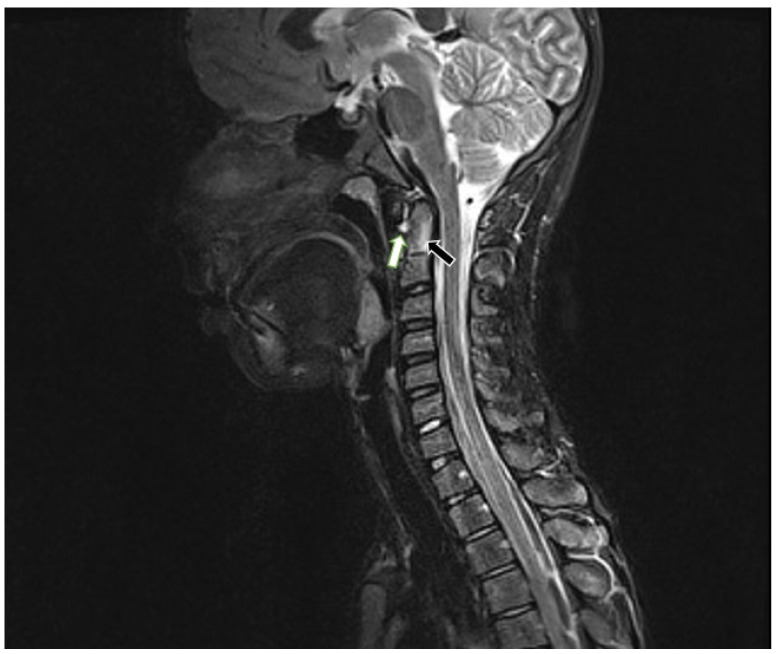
Sagittal MRI, TIRM T2w sequence in a 12-year-old boy diagnosed with enthesitis-related arthritis shows BME in the dens (black arrow) and atlantoaxial effusion (white arrow). MRI—magnetic resonance imaging, TIRM T2w-turbo inversion recovery magnitude T2 weighted, BME—bone marrow edema.
